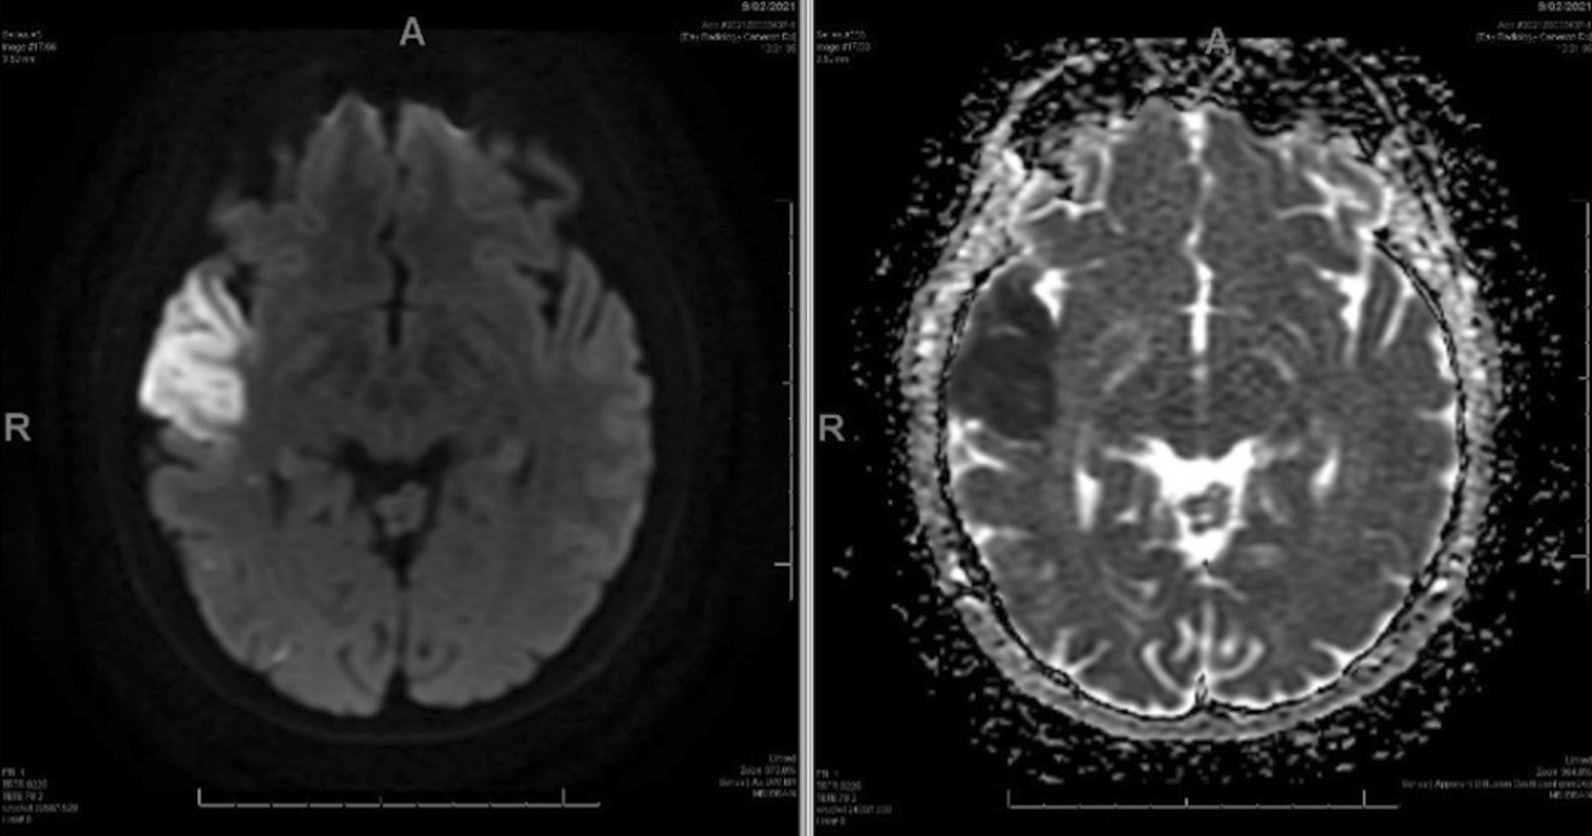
MRI brain at initial presentation
